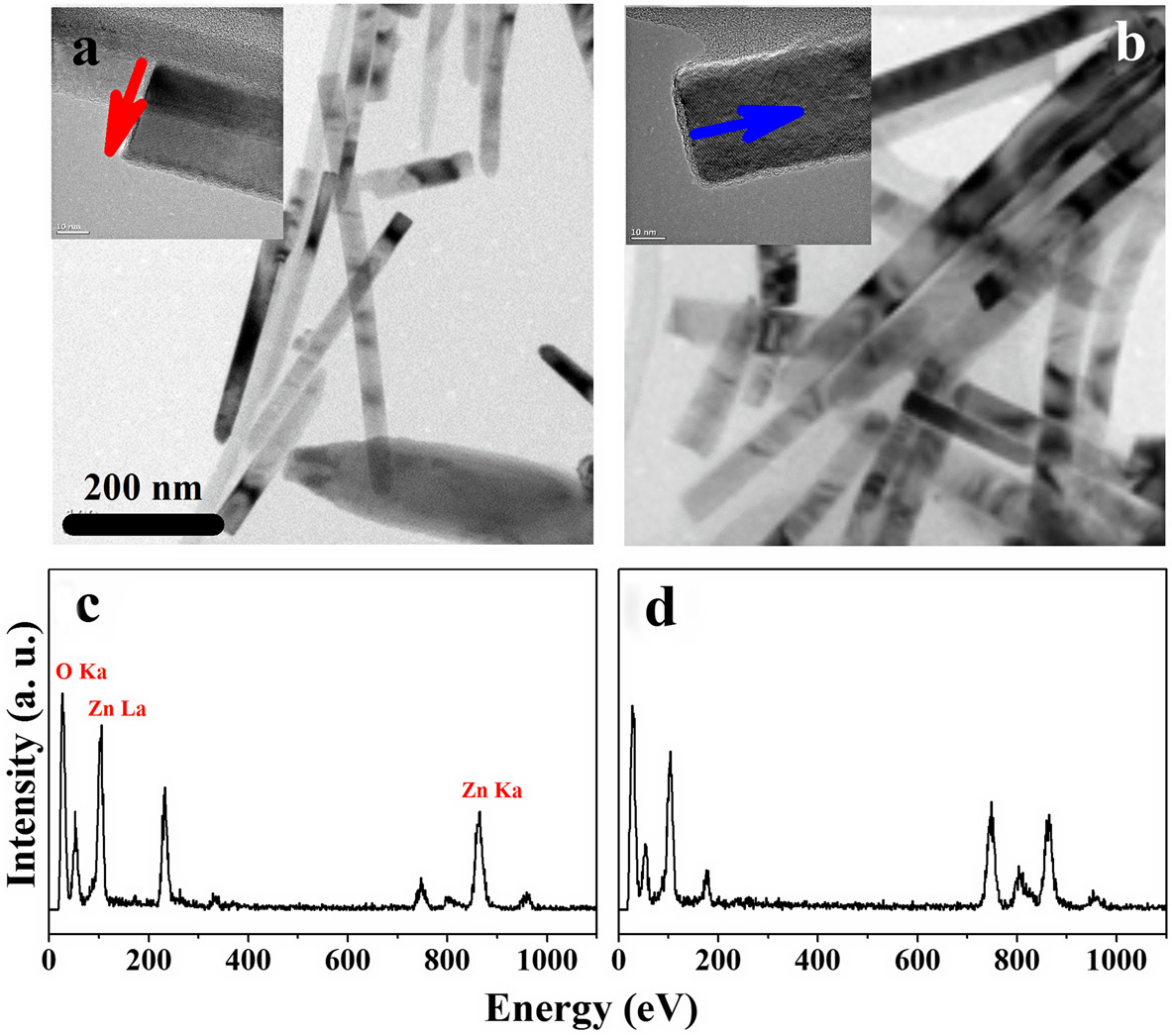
TEM images and the corresponding EDX spectra of ZnO nanorods grown for 4 h: a and c on the Si(001) wafer and b and d on the FTO glass substrate. We obtained these images and spectra of the nanorods after separating them from their respective substrates by using an ultrasonicator
